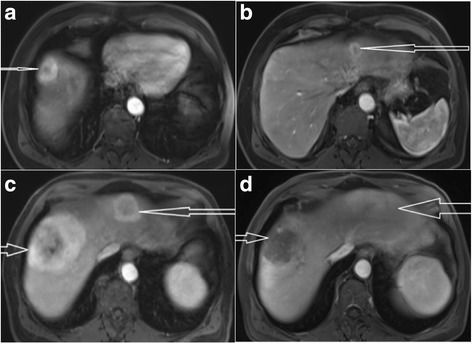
MRI abdomen T2-hyperintense signal. a & b showing multiple metastatic lesions to the liver before the initiation of immunotherapy. c showing progression of the disease after 4 cycles of nivolumab. The largest lesion was 5.5 cm in the right hepatic lobe. d showing a mixed response after 4 cycles on ipilimumab/nivolumab
